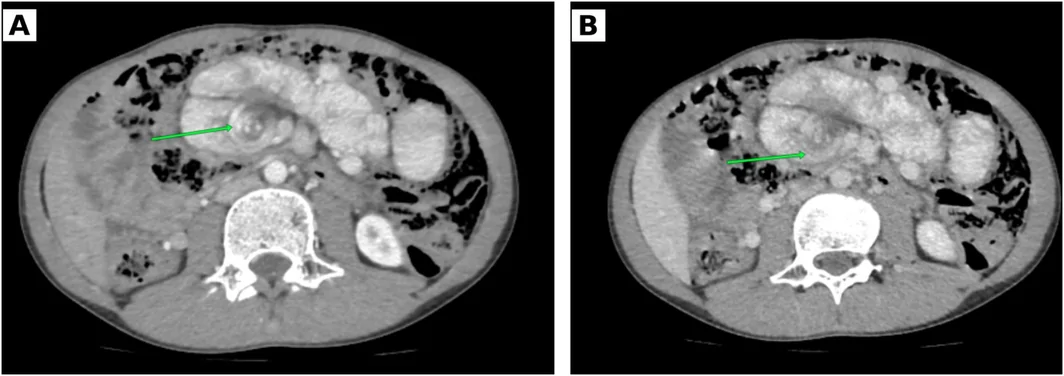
Axial post‐contrast abdominal CT scans. (A) The third part of the duodenum (arrow) failing to cross the midline. (B) The fourth part of the duodenum (arrow) also confined to the right side, with associated twisting and abnormal swirling of adjacent mesenteric fat, consistent with midgut volvulus.
